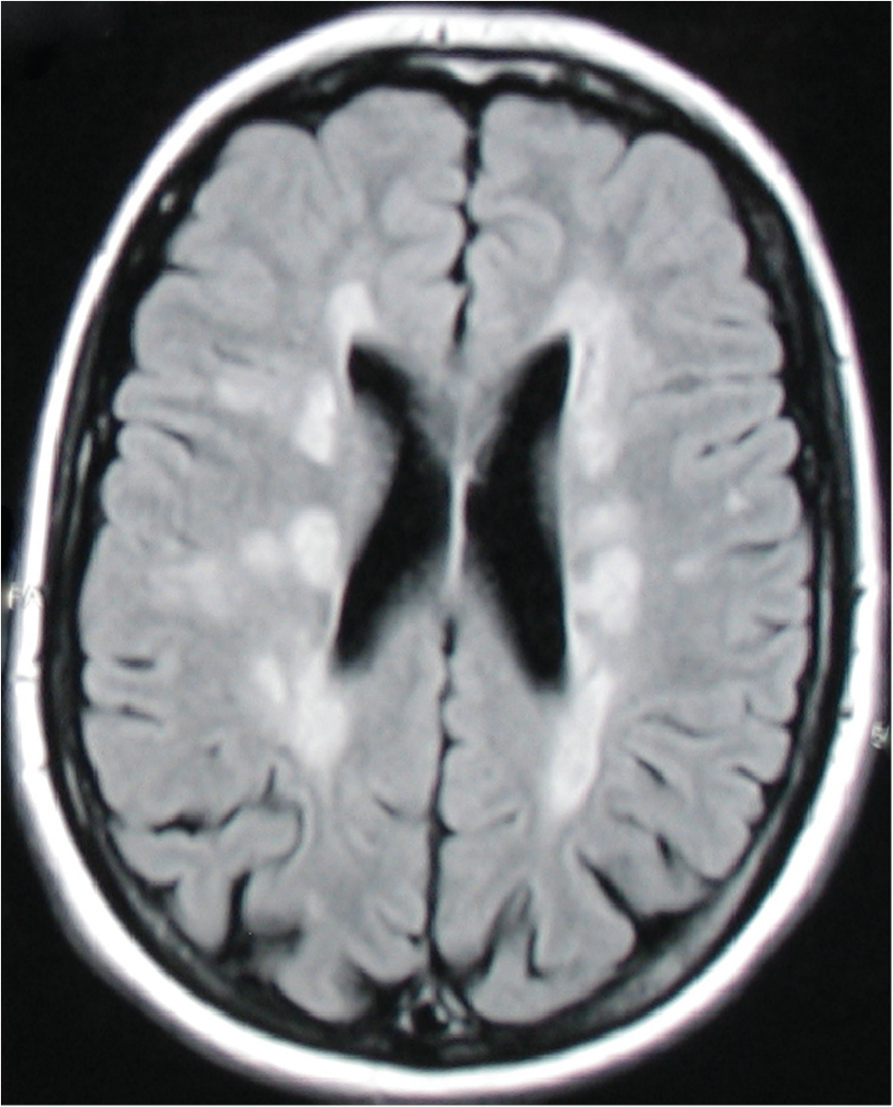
Brain MRI abnormalities in an anti-MOG antibody-positive patient. Axial T2-FLAIR (fluid-attenuated inversion recovery) brain MRI of a MOG-seropositive NMO patient 31 years after disease manifestation with combined optic neuritis and transverse myelitis showed multiple abnormalities fulfilling Barkhof criteria and resembling multiple sclerosis lesions.
